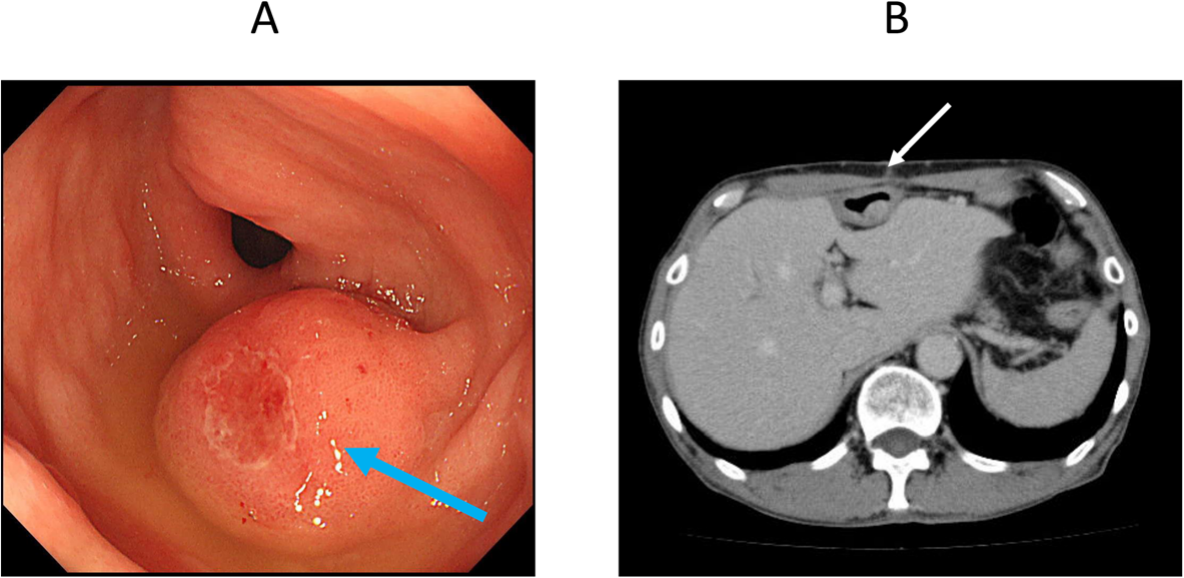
a EGD before chemotherapy. Submucosal tumor in the pyloric part of the gastric tube (blue arrow). EGD, esophagogastroduodenoscopy. b CT before chemotherapy. A 7-mm tumor (white arrow) located in the gastric tube. CT, computed tomography
